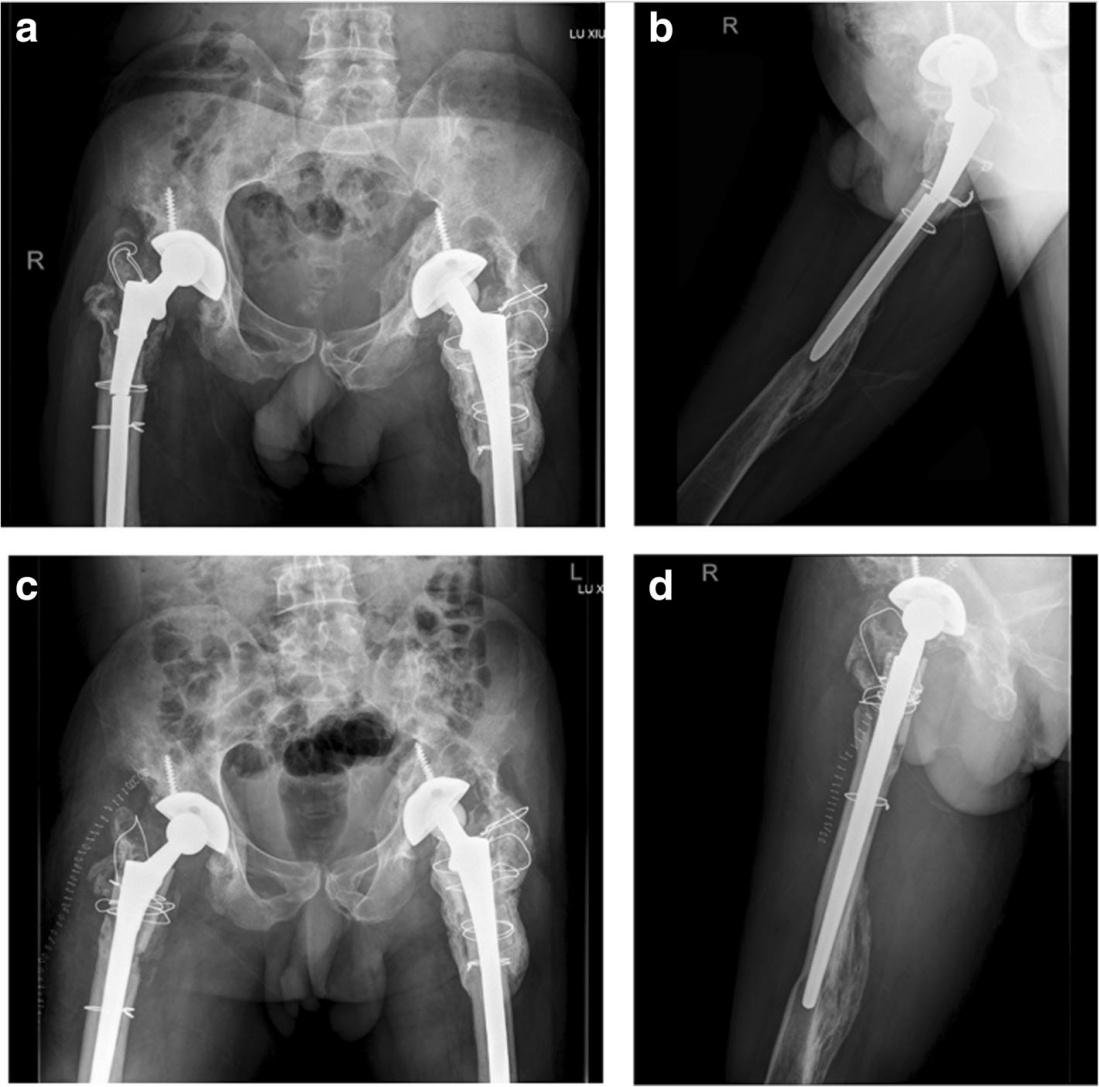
a and b: Pre-operative AP radiographs taken in September 2017 showing the right femoral stem fracture. c and d: Radiographs taken after revision of the right hip
